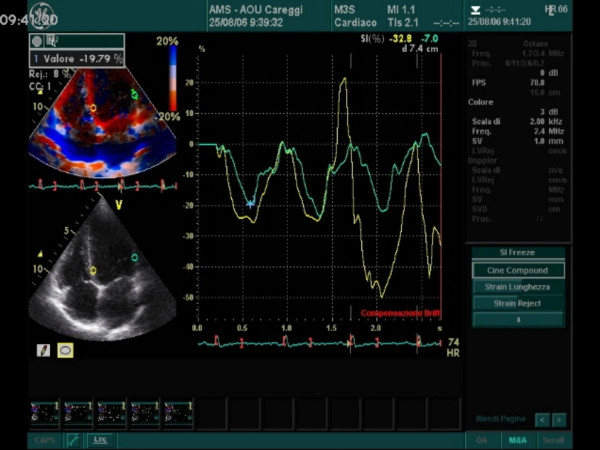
Systolic peak strain analysis at rest with Tissue Doppler : the panel shows left ventricle interventricular septum (IVS) and lateral wall (LW) values in basal segments.
